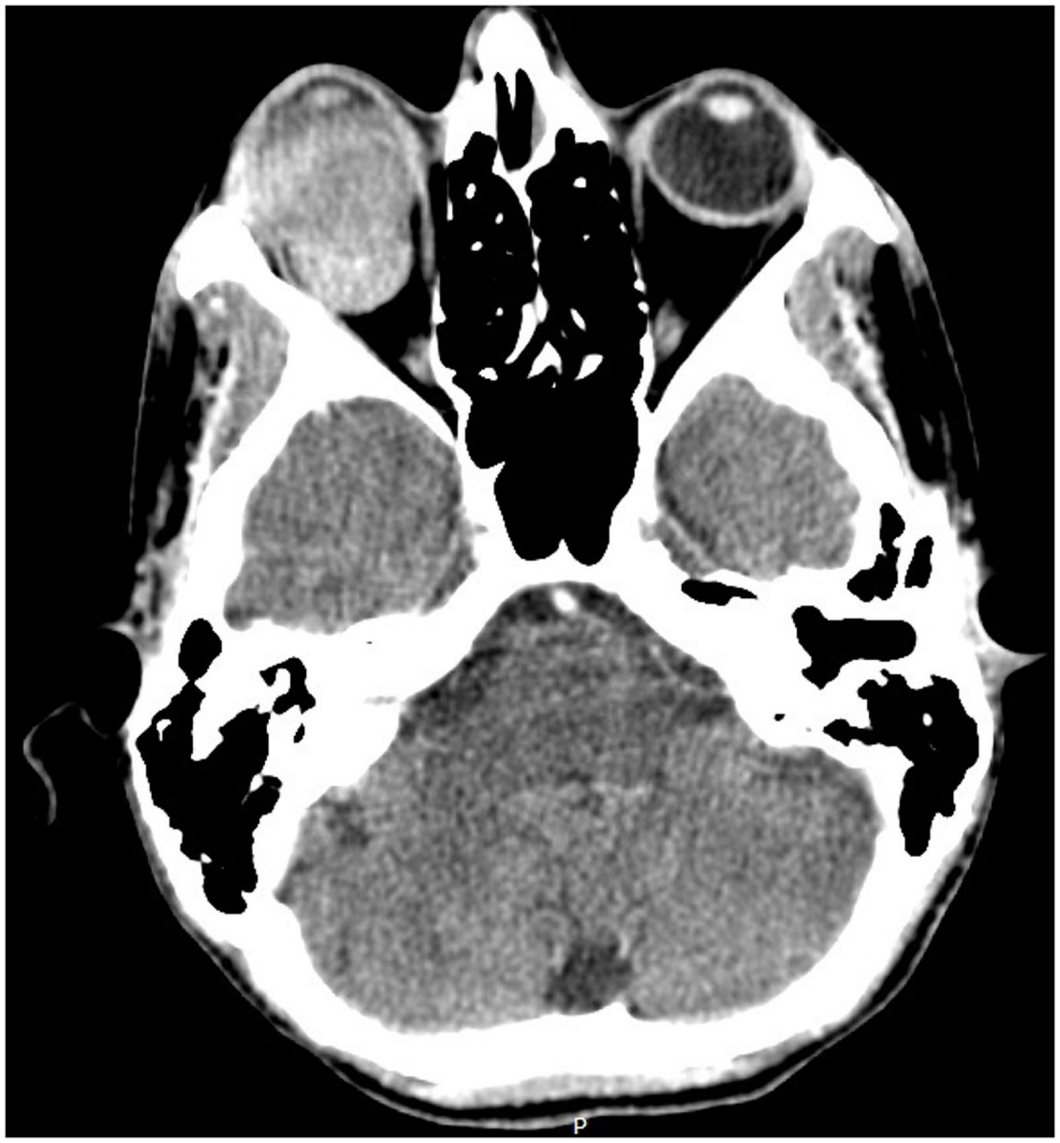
Orbital CT with the expansive lesion in the right eye.
